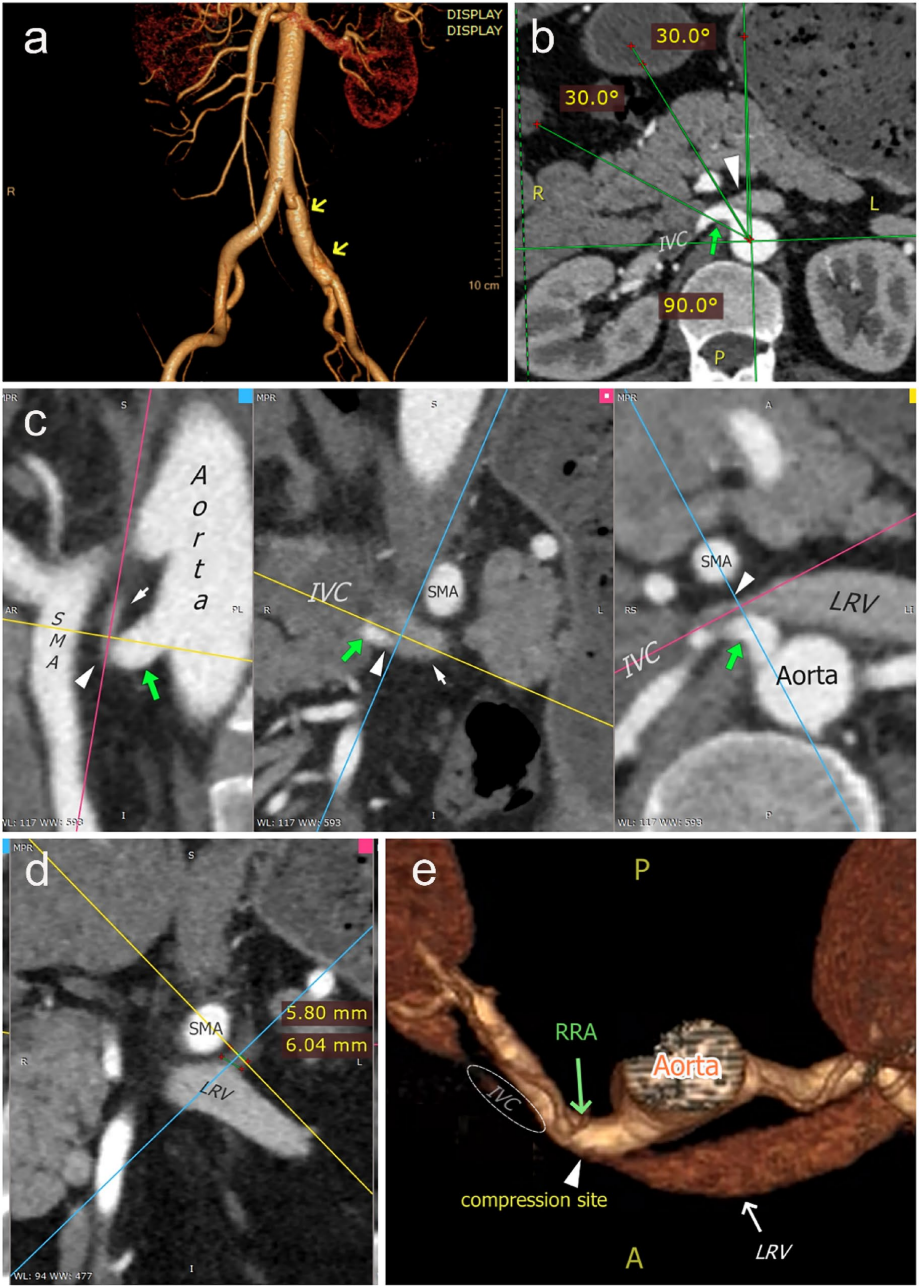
CT imaging showed a notable RRA-sourced LRV compression in a 48-year-old male with isolated iliac dissection (arrows in a). The initial segment of RRA (green arrows in b, c, and e), arising from an 11 o’clock direction (b), transversed behind and compressed (white arrowheads in b, c, and e) the ending segment of the LRV, where it joins the IVC. A dilated adrenal vein (the axes’ intersection in d) was visualized and measured. SMA, superior mesenteric artery; short-shafted white arrows (without text), LRV; other legends are the same as in Fig. 1
